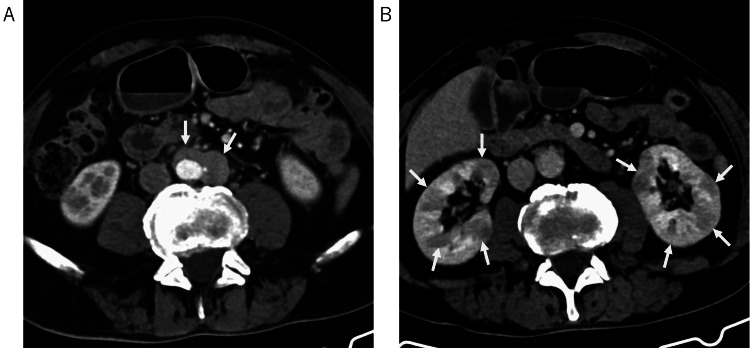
Abdominal findings on CT. Mass-like soft tissue is observed around the aorta (A). Multiple poorly enhancing areas are present in both kidneys (B).
